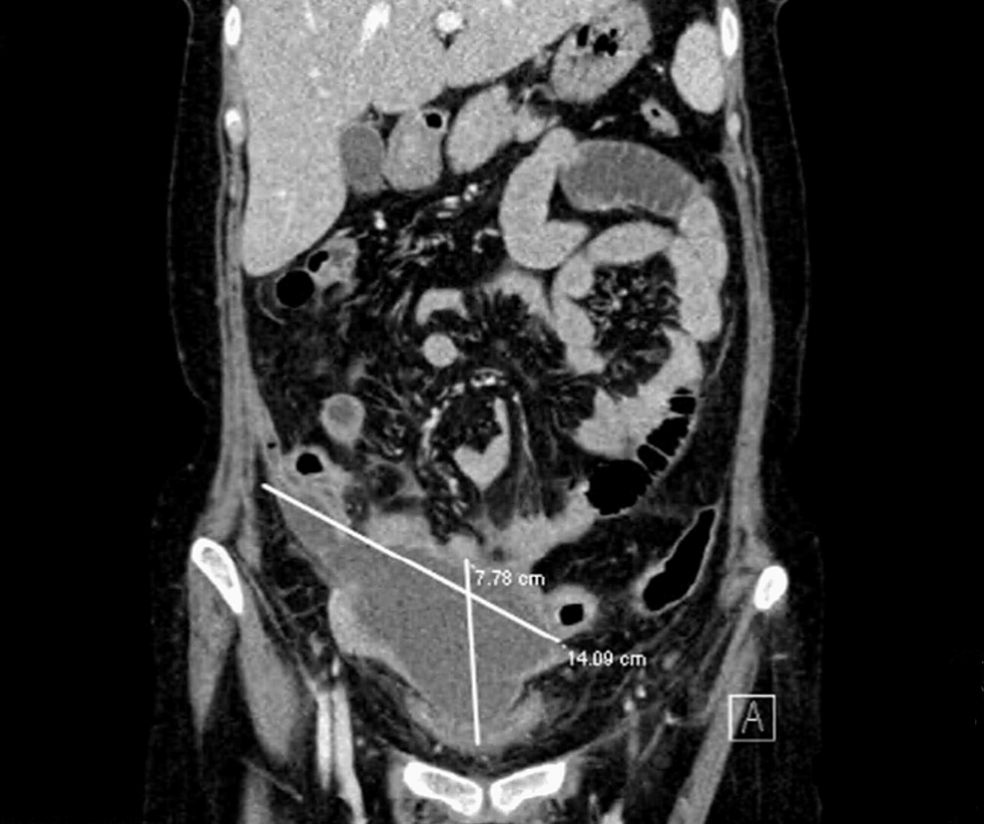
CT of the abdomen and pelvis showing a large irregular pelvic collection with rim enhancement
